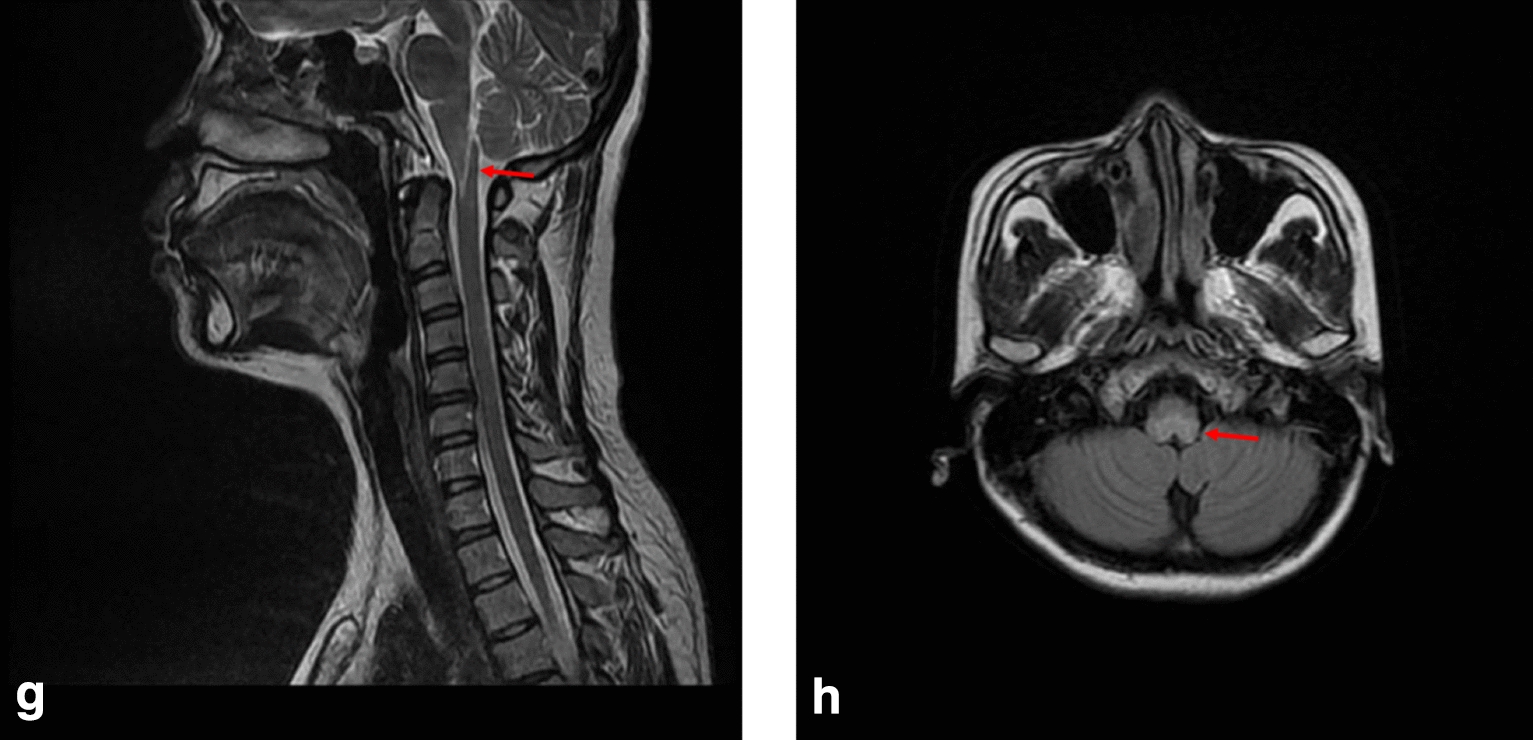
MRI T2 sequence of case 4 showing hyperintense in medulla oblongata (highlighted by arrows) in the g sagittal planes and h transverse planes
